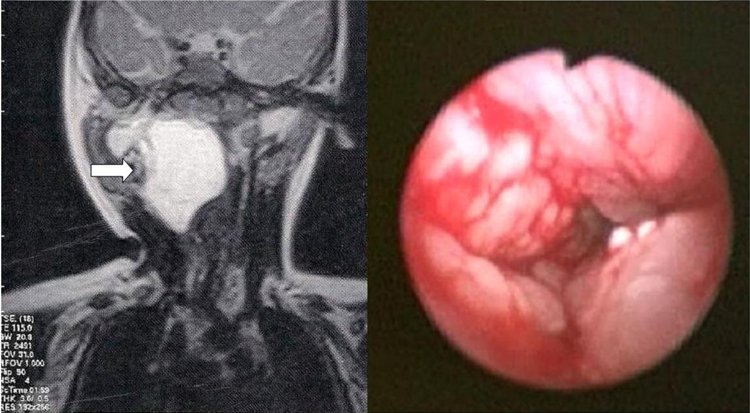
Magnetic resonance imaging, showing lesion in the retropharynx to the right (arrow). Endoscopic image of the upper airway (picture on the right).
